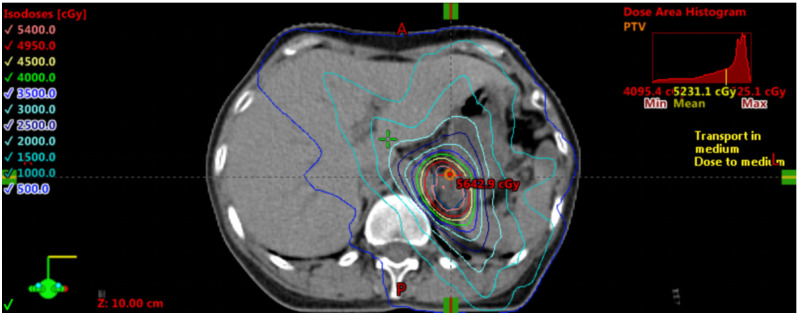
Radiotherapy plan dose distribution displayed on CT (lung window).
